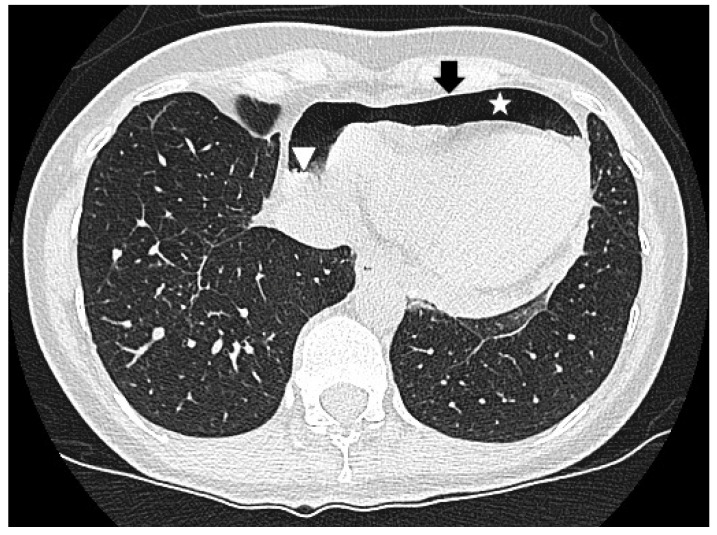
Chest computed tomography demonstrating air (star) within the pericardium (arrow) as well as an air–fluid level (arrow head), confirming pneumopericardium.
